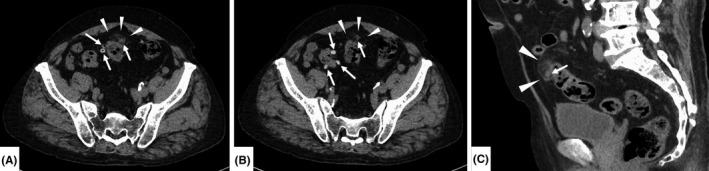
(A, B) Axial view. (C) Sagittal view. Thoracic and abdominal computed tomography without contrast enhancement shows multiple diverticula between the ascending colon and sigmoid colon (arrows). One of the diverticula near the sigmoid colon and adipose tissue around it show an area of high attenuation (arrowheads), suggesting a diagnosis of diverticulitis of the colon
